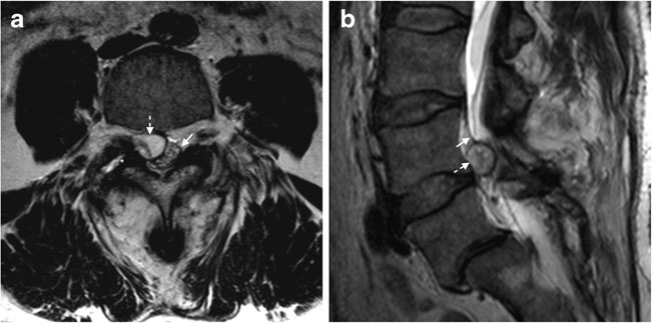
a, b. Lumbar facet synovial cyst in a 50-year-old man with a history of spinal surgery due to spondylolisthesis 20 years earlier, presenting with low back pain. Axial (a) and sagittal (b) T2-weighted images show a mildly hyperintense extradural rounded lesion (dashed arrows) arising from the right L4/L5 facet joint, which presents marked degenerative changes and fluid (asterisk). Note the compression of the thecal sac, displaced posteriorly (arrow in b) and to the left side (arrow in a). *Case courtesy of Dr. Carlos Casimiro. Neuroradiology Department, Centro Hospitalar de Lisboa Norte. Lisbon, Portugal*
